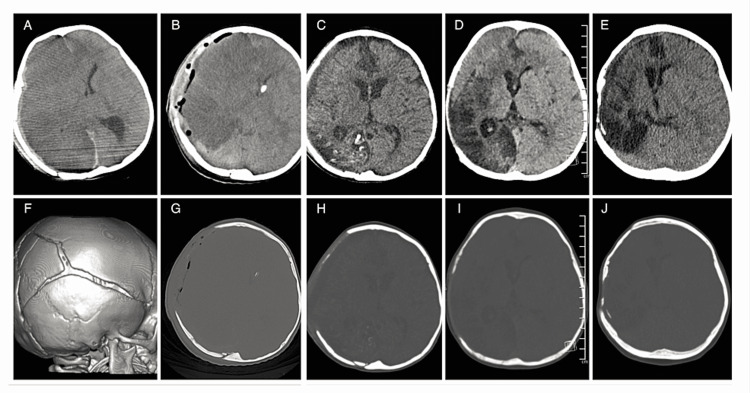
Non-contrast head CTs at successive time intervals pre and post middle meningeal artery embolization (A) Preoperative axial non-contrast CT head demonstrates an acute right-sided subdural hematoma, large right-sided hemispheric infarction, and significant midline shift; (F) 3D reconstruction of the preoperative scan demonstrates multiple skull fractures crossing sutures. (B) Postoperative CT demonstrating craniectomy defect with left-sided ventricular drain placement. (C) CT obtained prior to discharge to inpatient rehabilitation facility. (D) CT obtained at three month outpatient follow-up demonstrating formation of the right frontal chronic subdural hematoma deforming the frontal lobe. There is extensive cortical and subcortical encephalomalacia of the right hemisphere. (E) Follow up CT obtained six months after MMA embolization demonstrating complete resolution of the right frontal chronic subdural hematoma. (G-J) Axial non-contrast CT head corresponding to same slices seen on (B-E) but with windowing optimized for bone to demonstrate progressive ossification over the craniectomy defect between each imaging interval.
